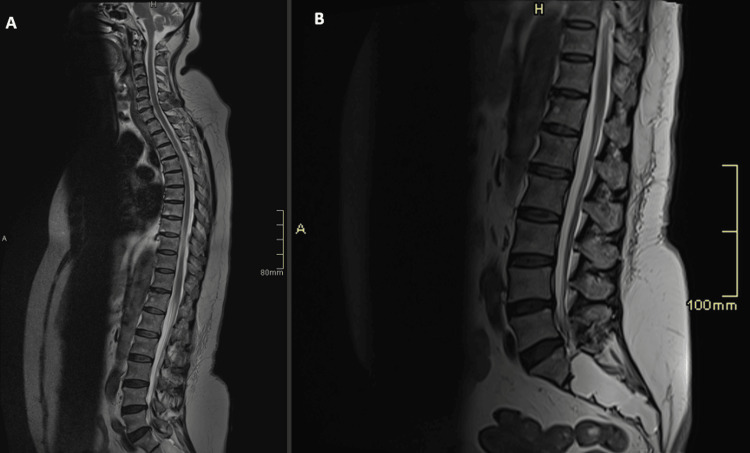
MRI whole spine and repeat MRI lumbar spine. Sagittal T2-weighted MR image of the whole spine, which was reported as a normal study (A). Sagittal T2-weighted MR image of the lumbar spine performed three weeks after the first study (A) demonstrated normal appearances and no discrete lesions. Incidentally, a large Tarlov cyst was seen in the sacral spinal canal, with no abnormal enhancement after administration of gadolinium (B).
